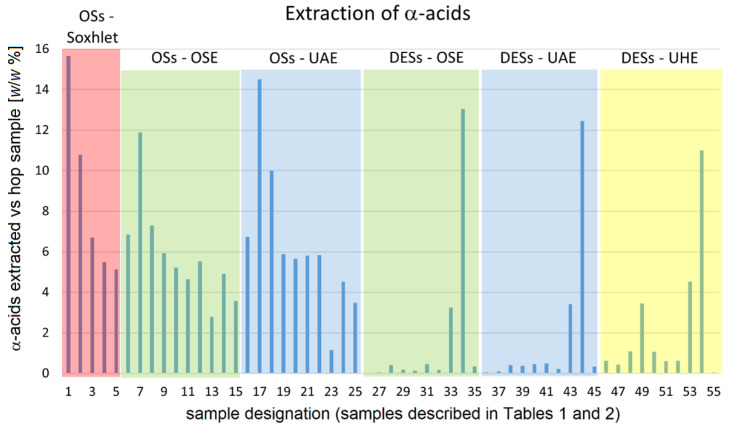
Quantity of α-acids extracted (reported as weight% of grinded hops pellets) as a function of both the extraction method and of the kind of extraction solvent used. Abbreviations: OSs-Soxhlet → organic solvents, Soxhlet extraction; OSs-OSE → organic solvents, orbital shaker extraction; OSs-UAE → organic solvents, ultrasound-assisted extraction; DESs-OSE → Deep Eutectic Solvents, orbital shaker extraction; DESs-UAE → Deep Eutectic Solvents, ultrasound-assisted extraction; DESs-UHE → Deep Eutectic Solvents, ultrasonic homogenizer extraction. Typical relative error in quantity of extracted α-acids is estimated to be around 3%.
